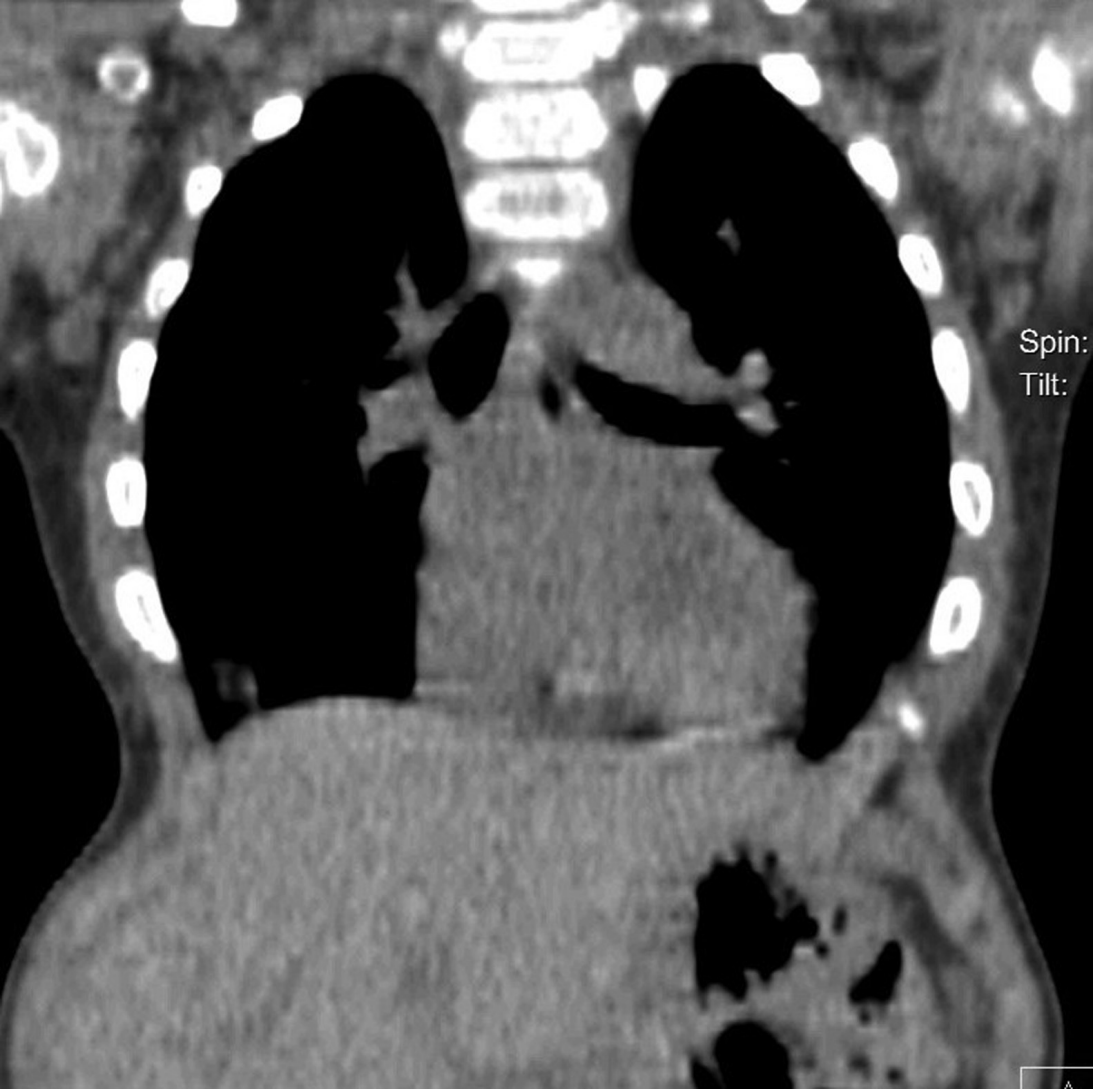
Computed tomography scan of the chest 5 months before the operation demonstrates the lung compression.
